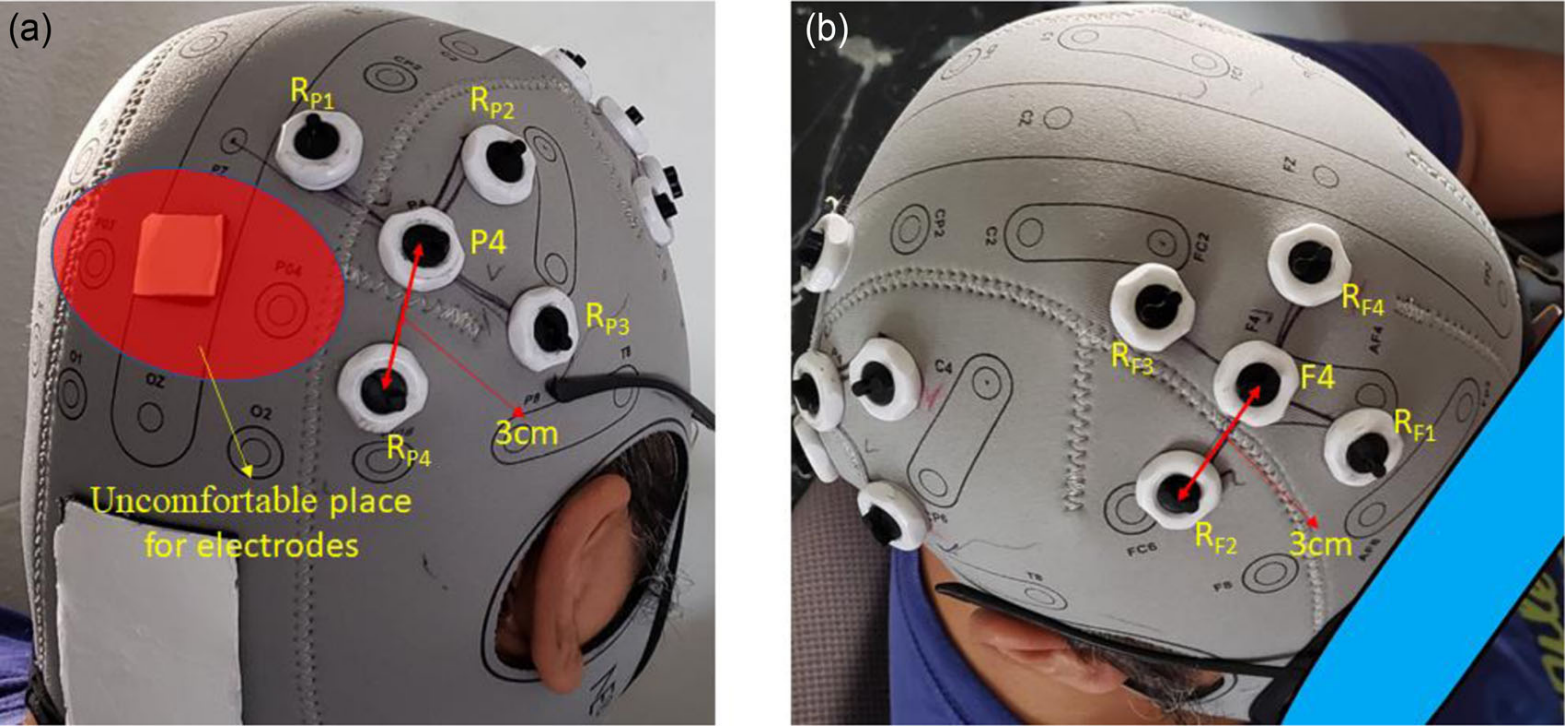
Overview of the frontoparietal montage with 10 HD electrodes. (a) Montage of five high‐definition (HD) electrodes on the parietal site with equidistant (3 cm) center and return electrodes. The red highlighted area is a subject uncomfortable area where we need to avoid placing the electrodes due to the constant head pressure when subjects lie down on the MRI table; (b) Montage of five HD electrodes on the frontal site with equidistant (3 cm) center and return electrodes. Red area indicates the area needs to be aware of for MRI scanning due to the pressure when subjects lie down on the MRI table.
